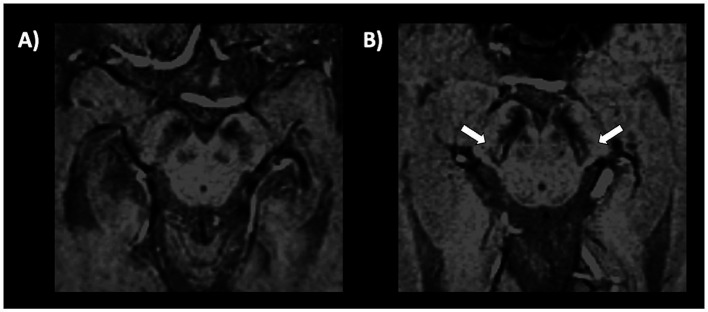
A 3T brain susceptibility weighted imaging (SWI) axial MR image showing the substantia nigra in a patient with Parkinson’s disease (A) and in a healthy subject (B). The substantia nigra in the left image has a homogeneous dark appearance bilaterally, and the nigrosome-1 is not visible. Conversely, in the image on the right side of the figure, a small hyperintense area corresponding to the nigrosome-1 is visible in the dorsolateral part of the substantia nigra, bilaterally (swallow-tail sign present, which is the normal appearance).
